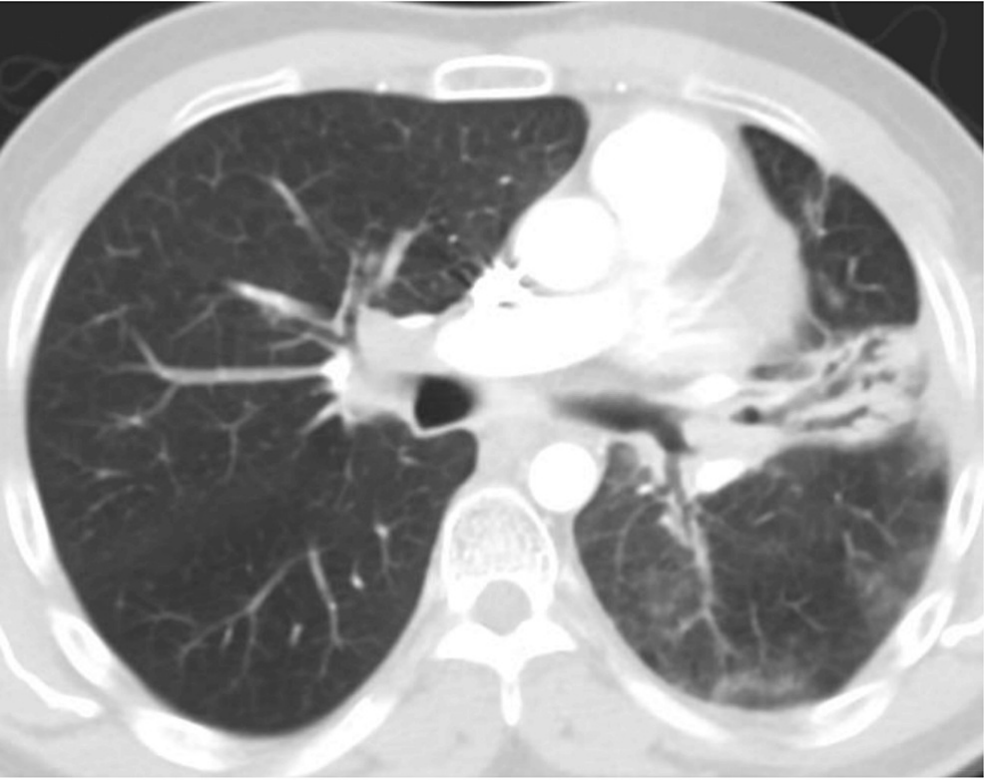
CT showing adenopathy at the hila has improved (June 12, 2012) CT: computed tomography
